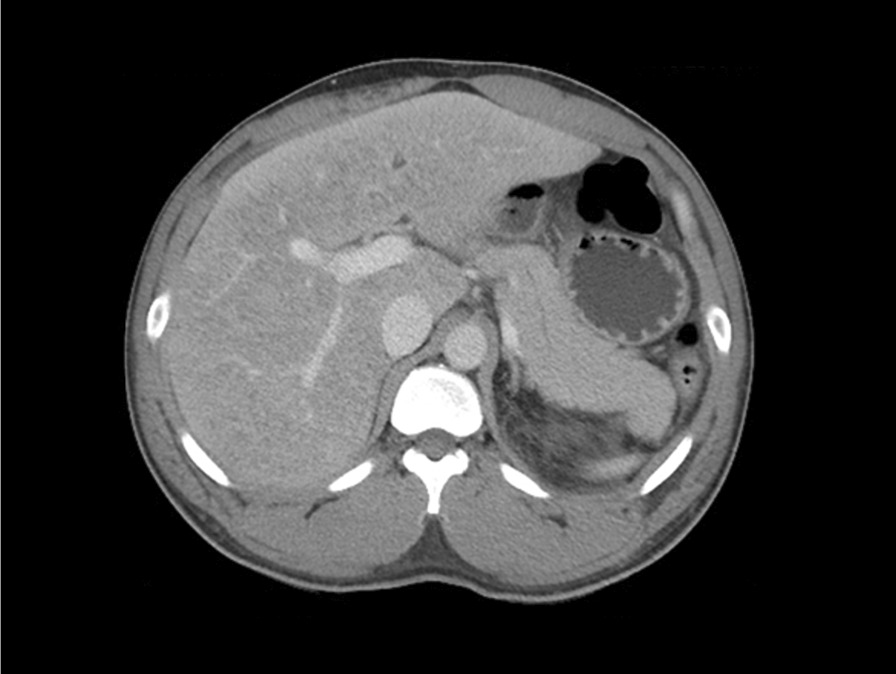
Abdominal computed tomography in the axial plane showing diffuse thickening and edema of the body and tail of the pancreas with peripancreatic fat stranding and no evidence of pancreatic necrosis
